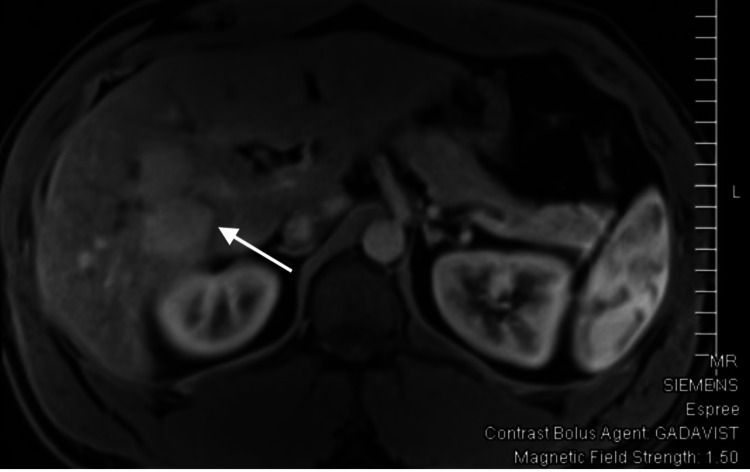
MRI abdomen (Case 1) showing numerous dominant, LI-RADS 5 lesions with arterial enhancement and washout as well as multiple arterially enhancing lesions with variable washout throughout the liver.
